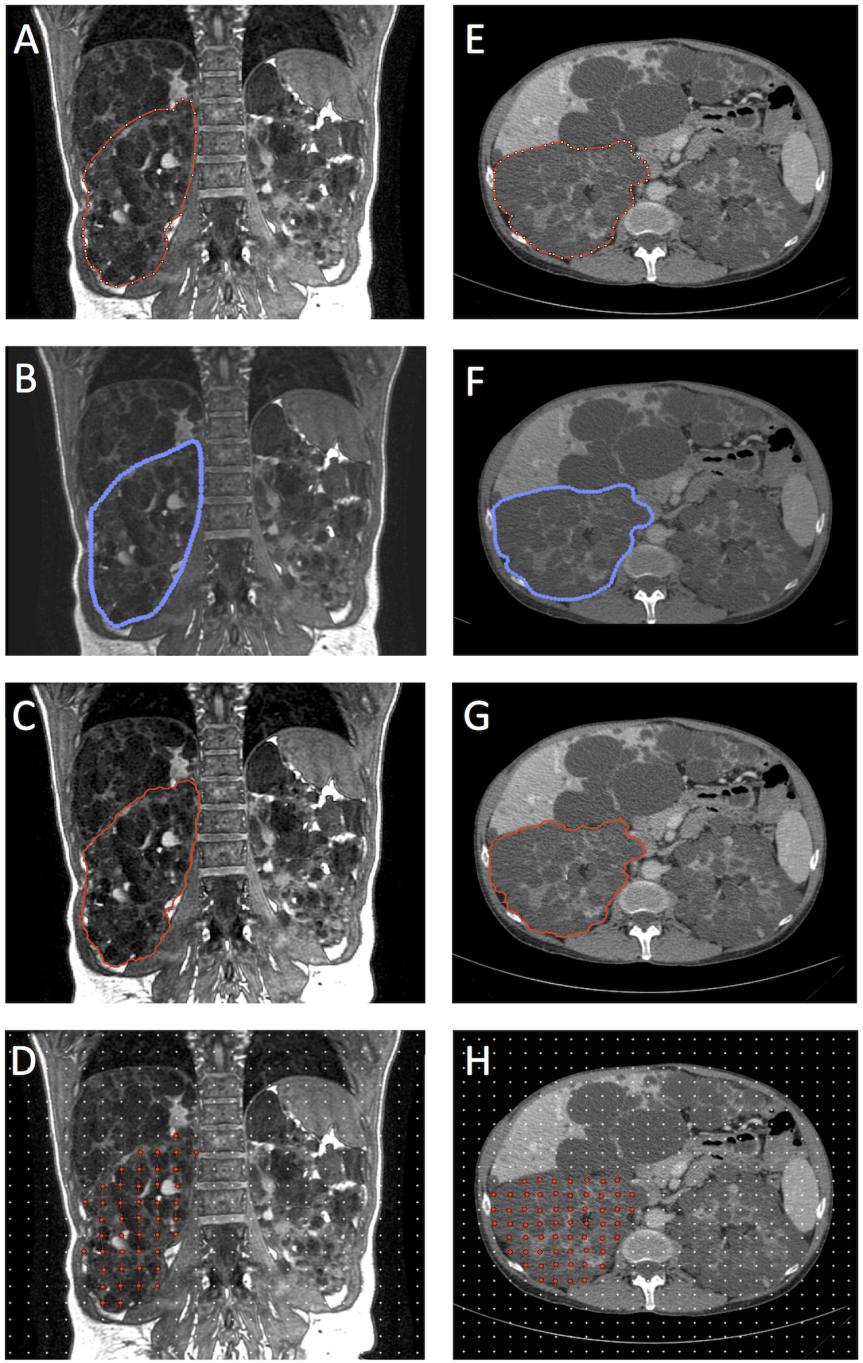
Representative images of polycystic kidney volume segmentations. Segmentation was performed on MRI (panels A-D) and CT image slices (panels E-H) by the expert operator using ImageJ polyline (A and E), Osirix free-hand (B and F), Livewire tool (C and G) and Stereology (D and H).
